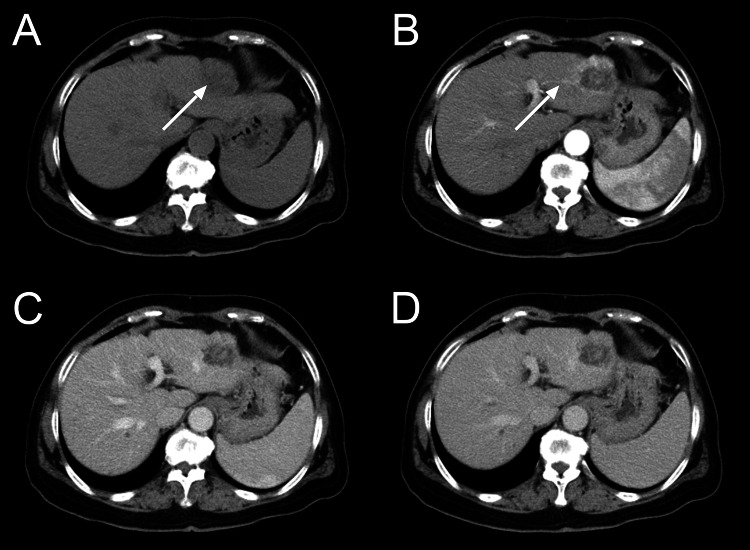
CT imaging of BAF. (A) An abdominal plain CT scan revealed a subcapsular solid-cystic mass with an obscure boundary (white arrow) in the liver segment II. The mass showed markedly heterogeneous enhancement in the (B) arterial phase, followed by wash-out in the (C) venous and (D) delayed phases. The arterial phase (B) also showed that the blood supply came from the left inter-hepatic artery and its branches. BAF: Biliary adenofibroma.
